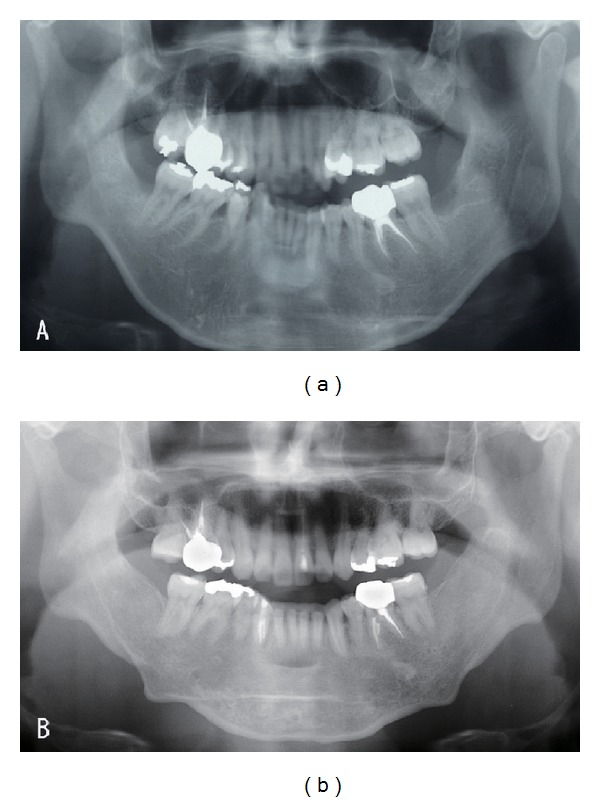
Orthopantomograph. (a) Pretreatment. (b) Posttreatment. Root resorption of the lower incisors is not observed.
